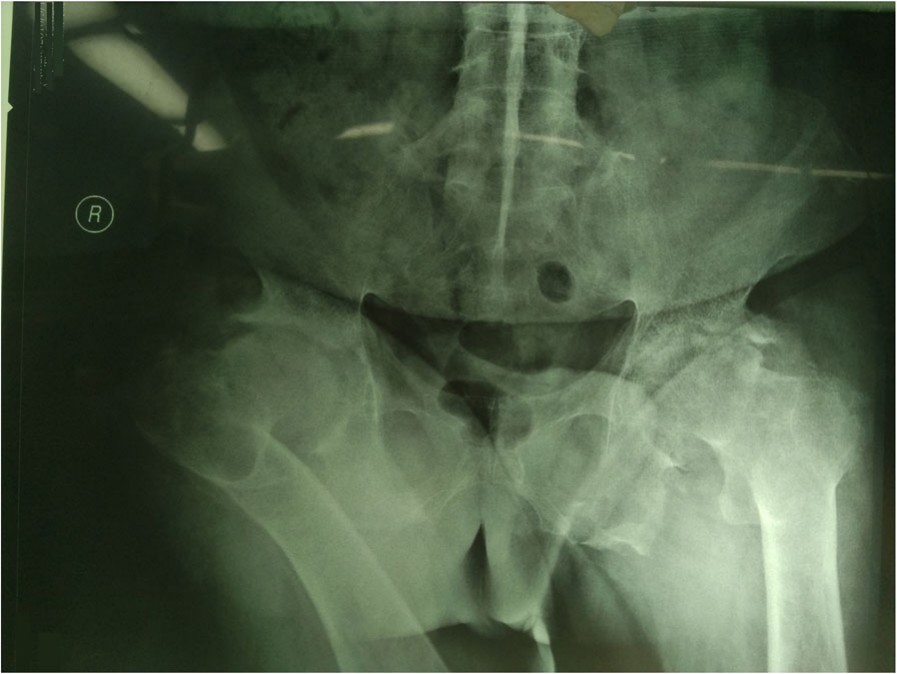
Radiograph of lumbo-sacral vertebrae and pelvis shows bamboo like bony bridge, narrowed intervertebral space, fusion of both sacroiliac joints and extreme deformity of both hip joints
